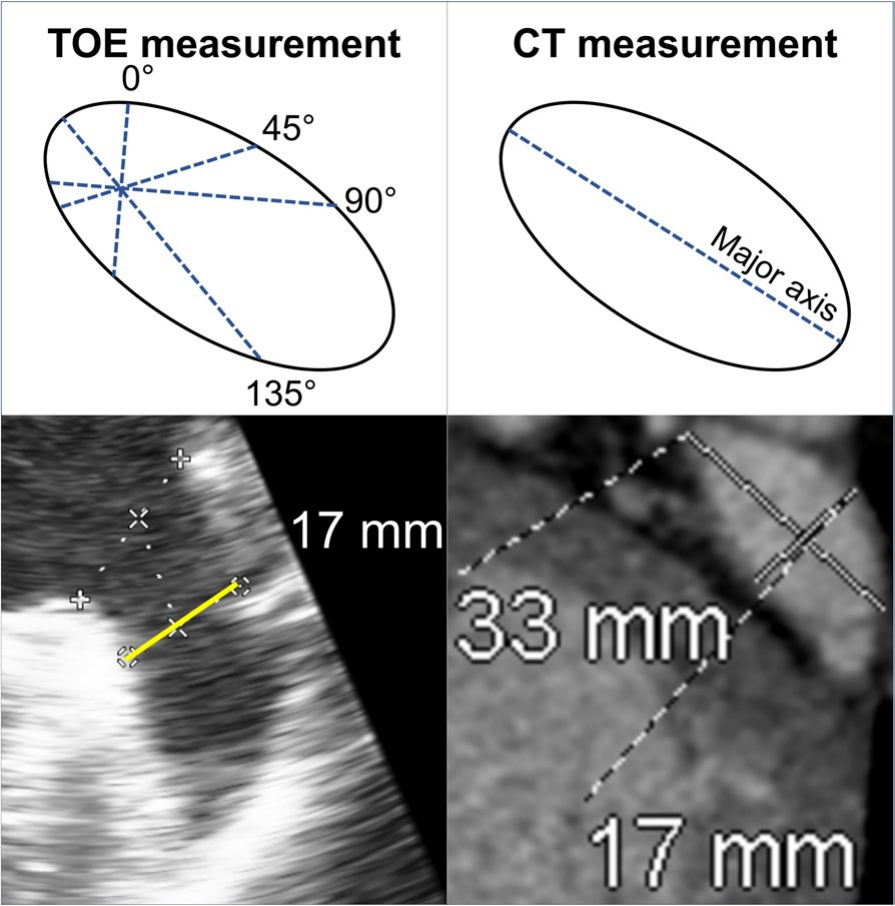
Comparison of transesophageal echocardiography (TOE) and computed tomography (CT) assessments of maximum landing zone diameter in the same patient. Despite measurements from multiple angles, the two-dimensional assessment by TOE may miss the major axis of an eccentric oval-shaped left atrial appendage, resulting in underestimation of the landing zone
